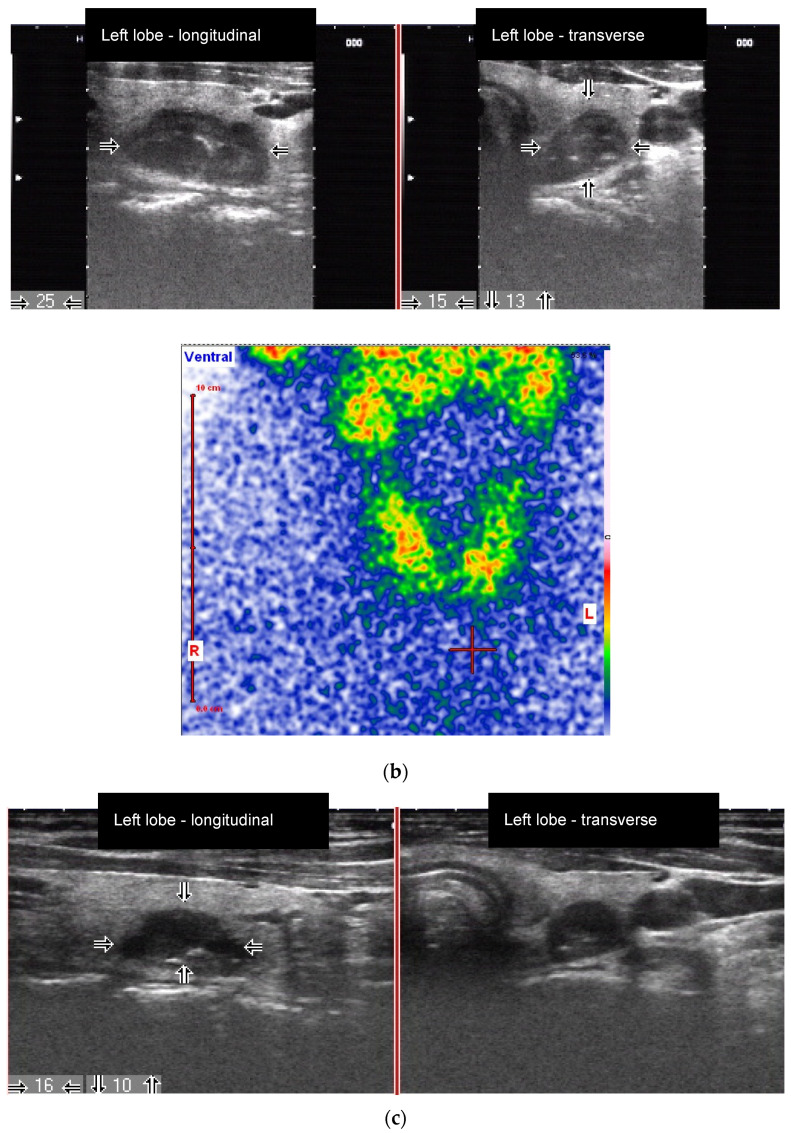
Male patient with an autonomously functioning thyroid nodule (AFTN) (scintigraphy with ^99m^Tc-pertechnetate) in the left thyroid lobe (25 × 27 × 36 mm; nodule volume 12.2 mL, arrows). (a) Before radioiodine therapy (RIT) (2016, TSH value 0.06 mU/L, no thyroid medications) with 1033 MBq (206 Gy due to reduced half-time at therapy). TIRADS classification: Kwak-TIRADS 4A (taller-than-wide shape); EU-TIRADS 5 (taller-than-wide shape); (b) The same patient 6 months after RIT (follow-up 1, FU1). The thyroid nodule size decreased to 15 × 13 × 25 mm (volume: 2.4 mL, arrows). Scintigraphy with ^99m^Tc-pertechnetate shows a non-hyperfunctioning area in the left lobe. TIRADS classification: Kwak-TIRADS 4C (predominantly solid composition, hypoechoic, microcalcifications); EU-TIRADS 5 (microcalcifications); (c) The same patient 12 months after RIT (TSH value 1.4 mU/L, Levothyroxine 50 micrograms/day). The thyroid nodule size at FU2 was 10 × 16 mm (arrows). TIRADS classification: Kwak-TIRADS 4C (solid composition, markedly hypoechoic, microcalcifications and macrocalcification); EU-TIRADS 5 (marked hypoechogenicity, microcalcifications).
